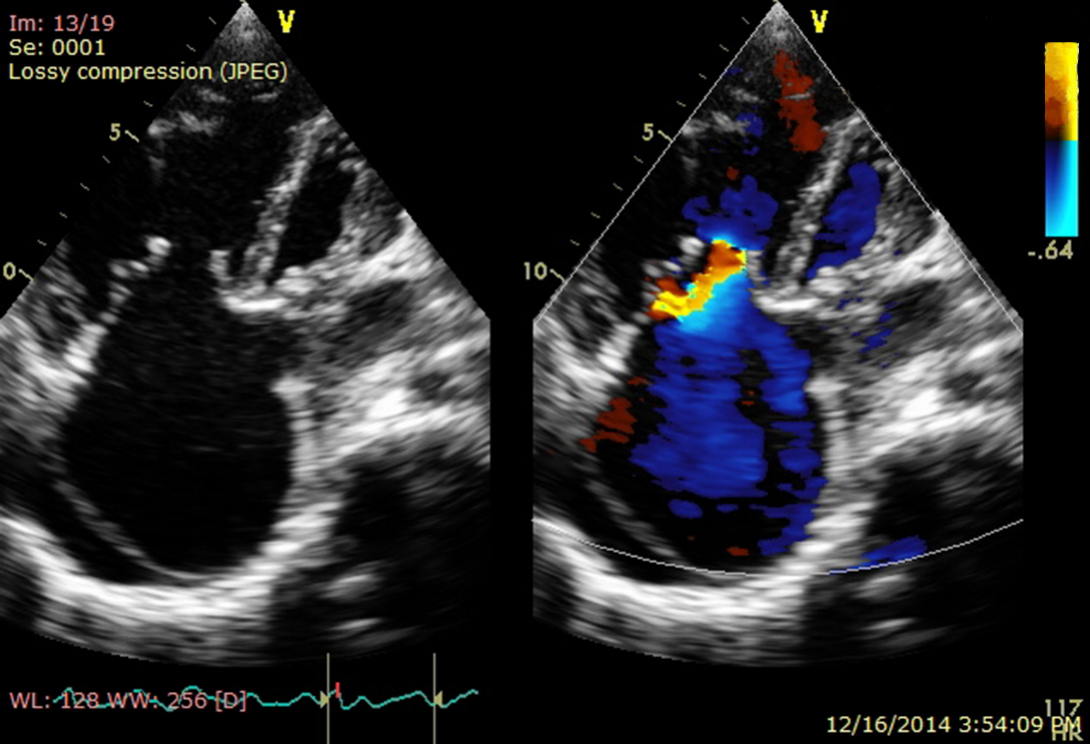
Transthoracic echocardiography, apical four‐chamber view, showing atrial septal defect sinus venosus type, dilatation of right atrium, right ventricle, and coronary sinus.
